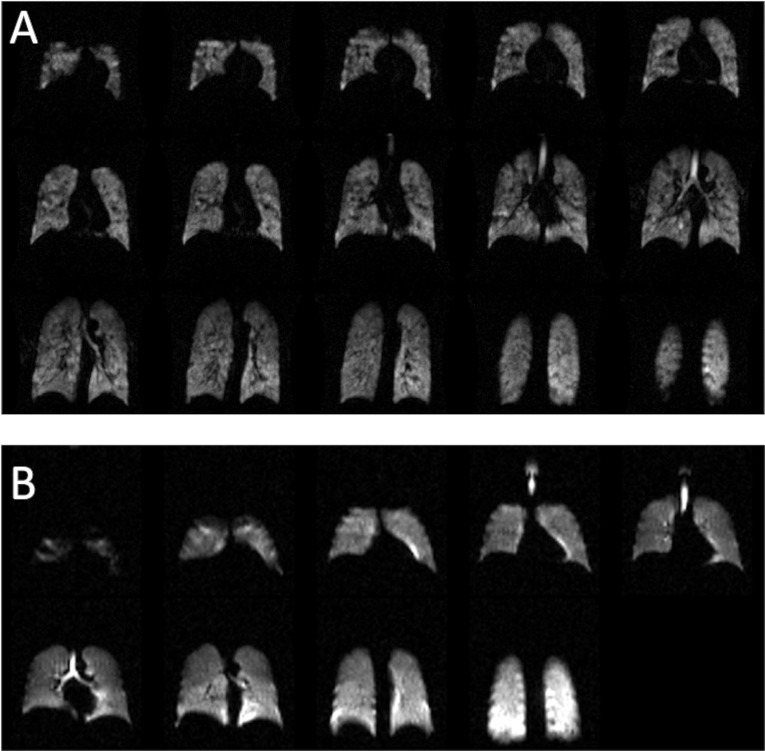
A: ^129^Xe lung ventilation MR images acquired on a 1.5 T clinical scanner at our imaging centre from a subject with asthma. Sequence details: repetition time msec/echo time msec, 6.7/2.3; field of view, 400×400 mm; matrix size, 80×80; slice thickness, 10 mm; flip angle, 10°; and bandwidth, 200 Hz/pixel. B: ^129^Xe lung ventilation MR images acquired on a 1.5 T clinical scanner at an external imaging centre the day after polariser transportation. Sequence details: volunteer, healthy male; repetition time msec/echo time msec, 6.9/2.3; field of view, 367×420 mm; matrix size, 56×64; slice thickness, 22 mm; flip angle, 10°; and bandwidth, 200 Hz/pixel.
